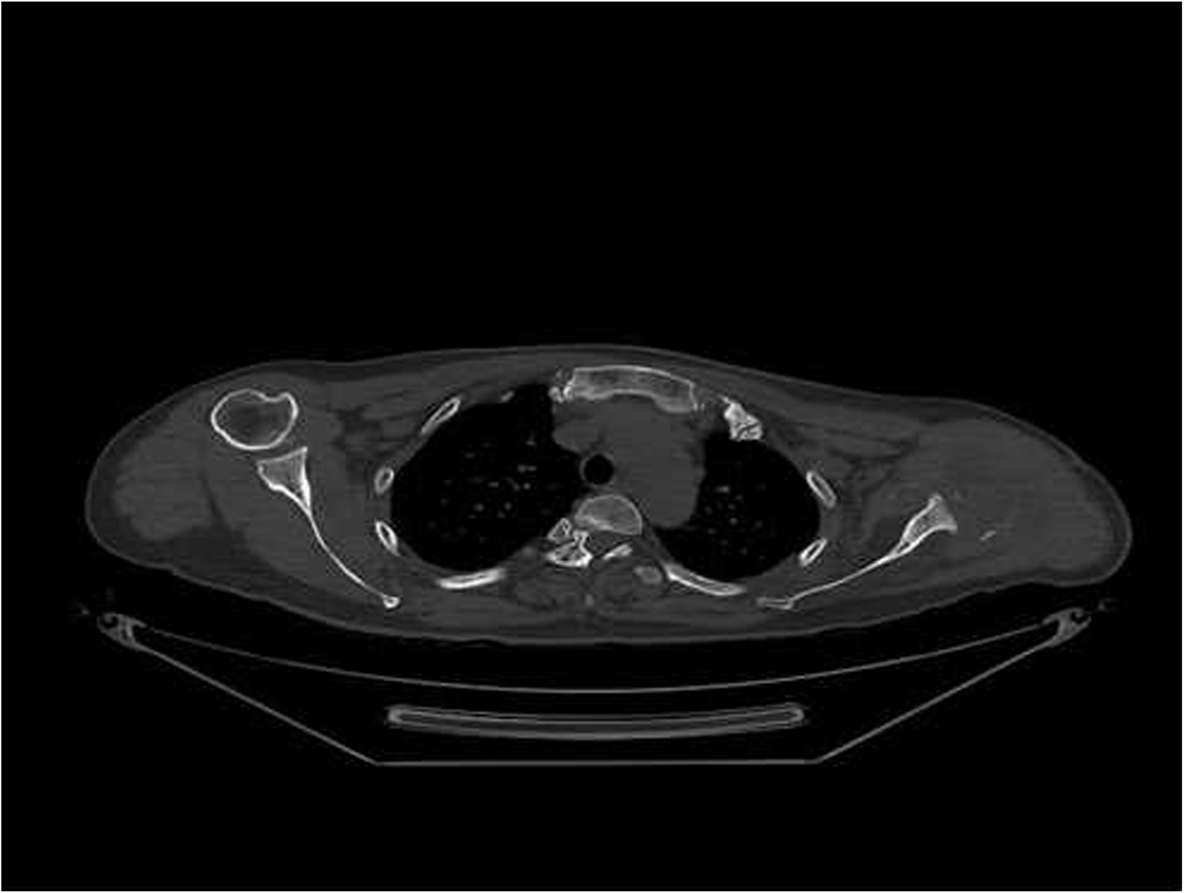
Computed tomography scan of the shoulder (coronal slice)
